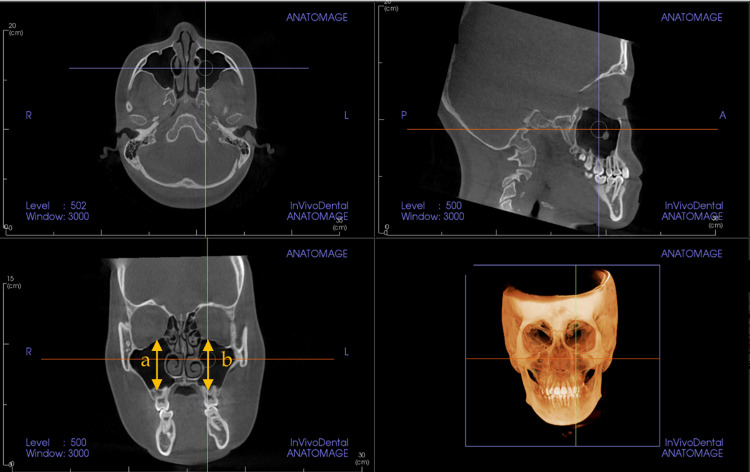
Cone-beam computed tomography (CBCT) images used to measure maxillary sinus height. The longest distance from the lowest point of the sinus floor to the highest point of the sinus roof was assessed using a coronal CBCT image. a: right maxillary sinus height; b: left maxillary sinus height
